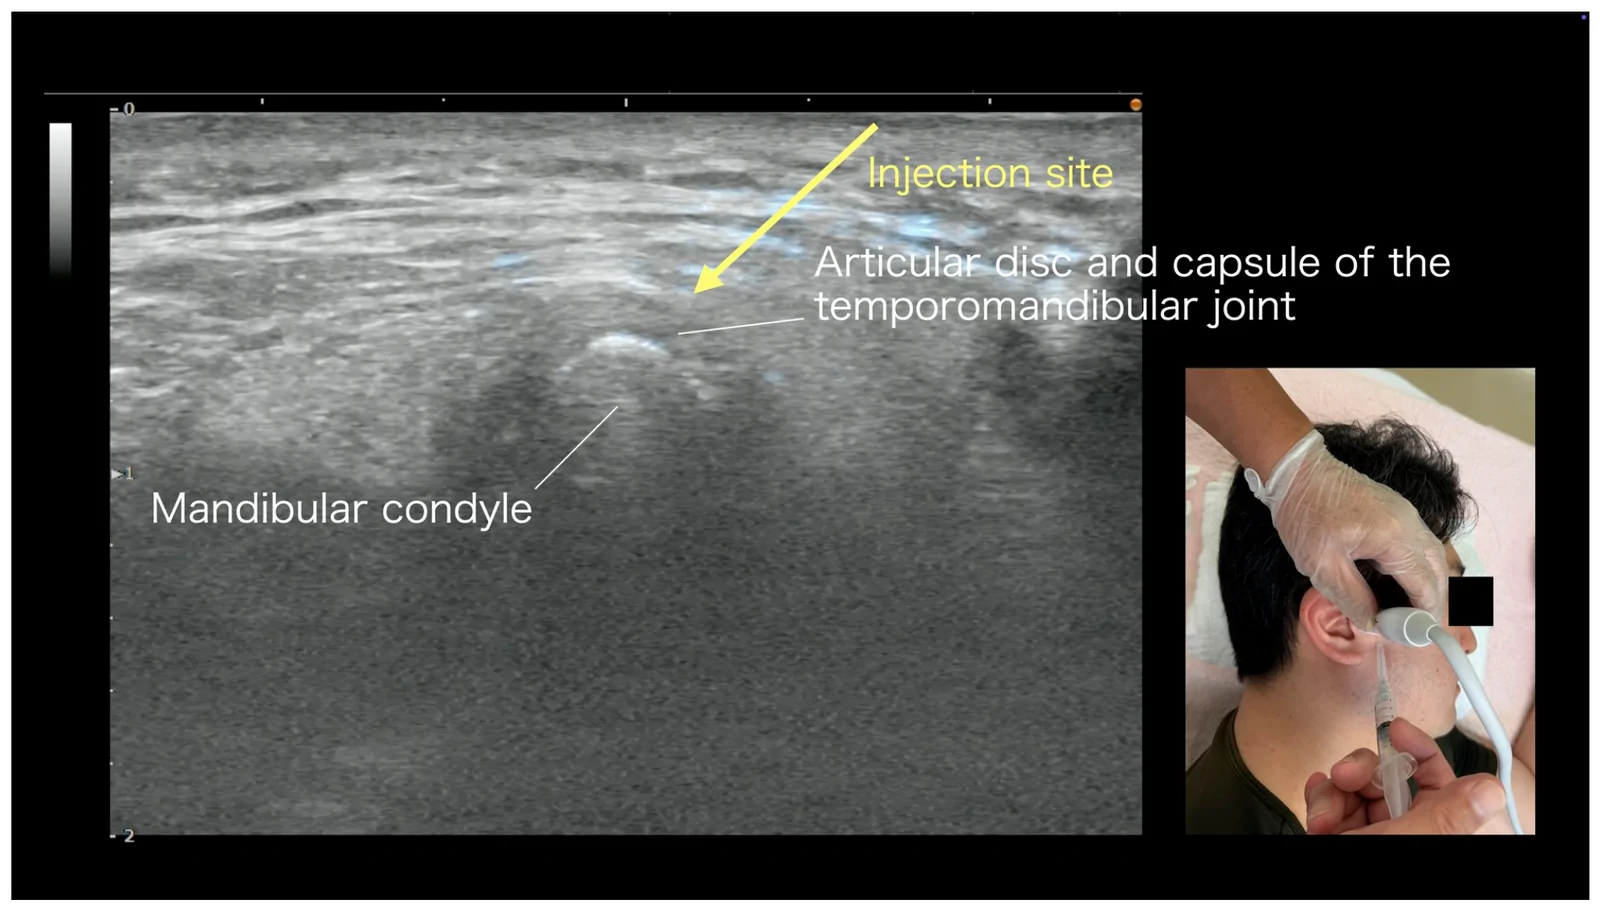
Temporomandibular joint capsule complex US-FHR (POINT 6). Ultrasound image: The mandibular condyle, articular disk, and joint capsule of the temporomandibular joint are identified. The yellow arrow (injection site) indicates the periarticular fascial space. The inset (bottom right) shows the patient position and probe placement.
